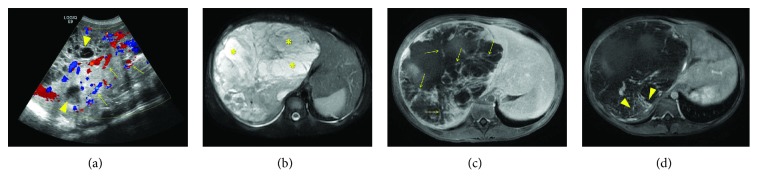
(a) Ultrasonography of the liver. Grayscale with Doppler image demonstrates a partially defined hepatic mass with multiple internal cystic foci (arrowheads) and increased intralesional vascularization (arrows). MRI of the abdomen with axial T2 fat saturation (b) and LAVA (liver acquisition with volume acceleration sequence) after intravenous contrast administration at 5 min (c) and 20 min (d) show a large heterogeneous mass within the right hepatic lobe. A predominant intralesional cystic/necrotic component (^∗^), multiple internal septations (arrows), and residual solid tissue (arrowheads) are seen.
